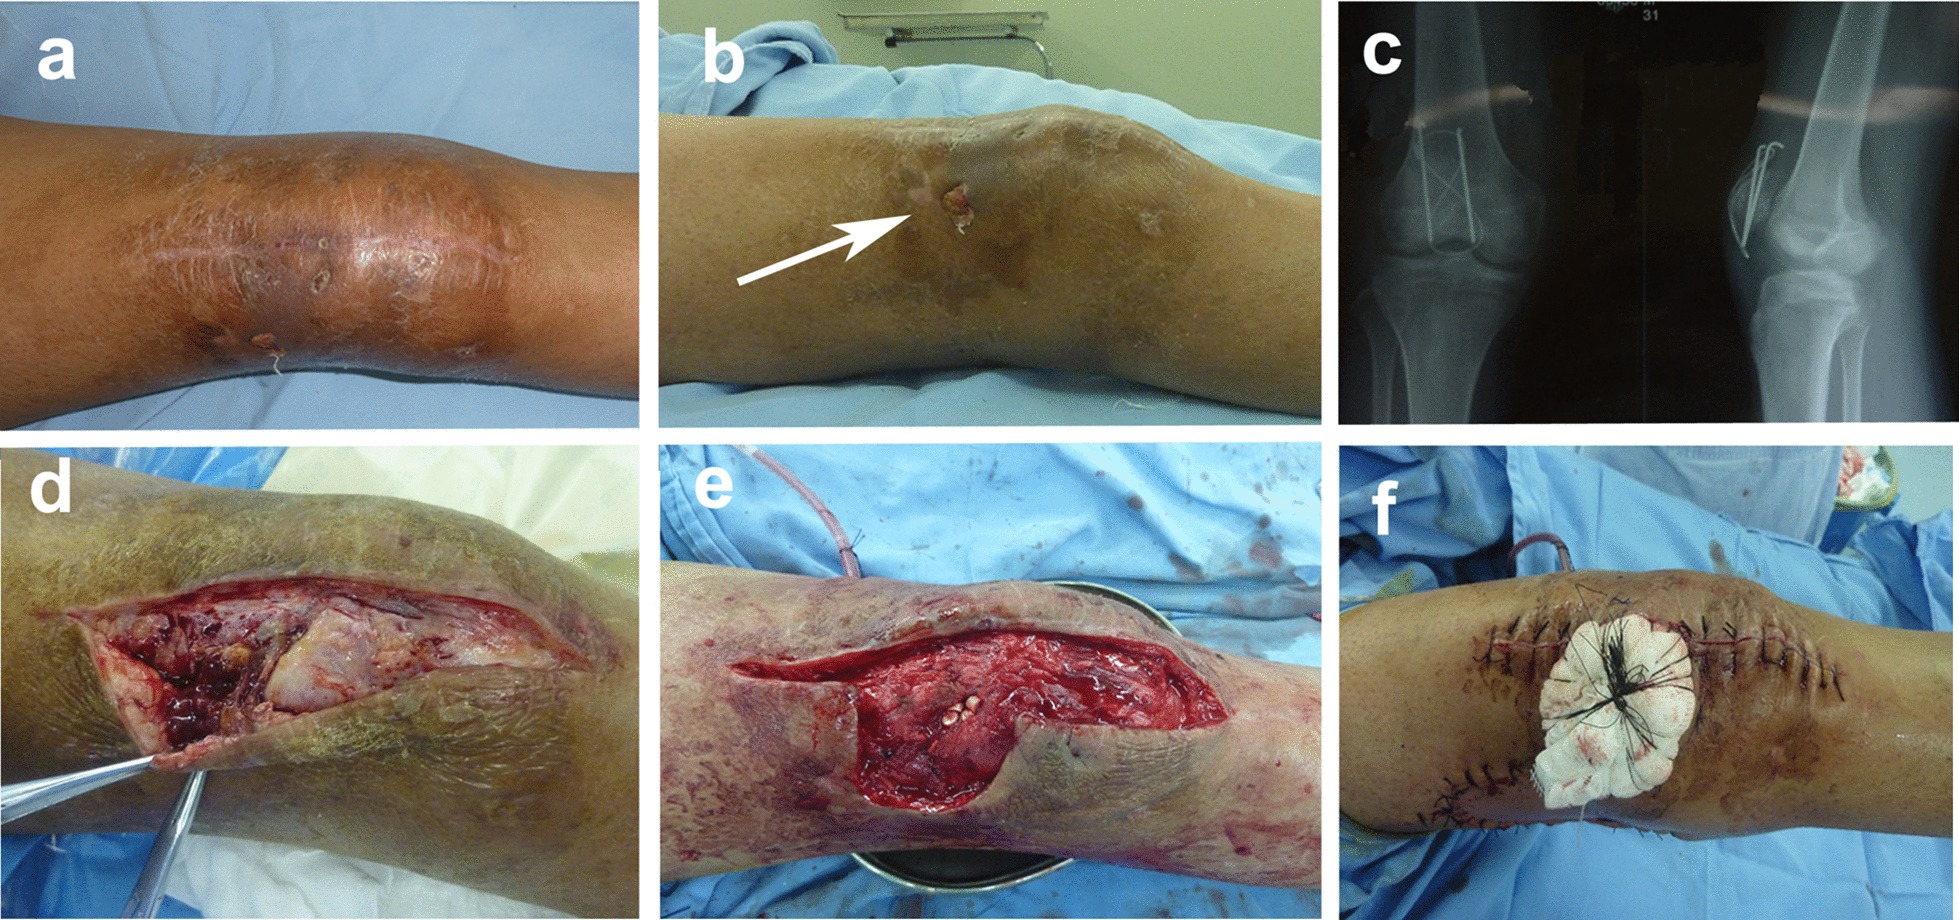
a, b Appearance of the right knee at the time of admission, and the arrow indicates infected sinus; c frontal and lateral radiographs of right patella at the time of admission; d infected bone and soft tissues were observed during operation; e intraoperative appearance after thorough removal of infected bone and soft tissues, and cavities were implanted with RBK beeds; f wound repaired by lateral gastrocnemius flap and skin graft
